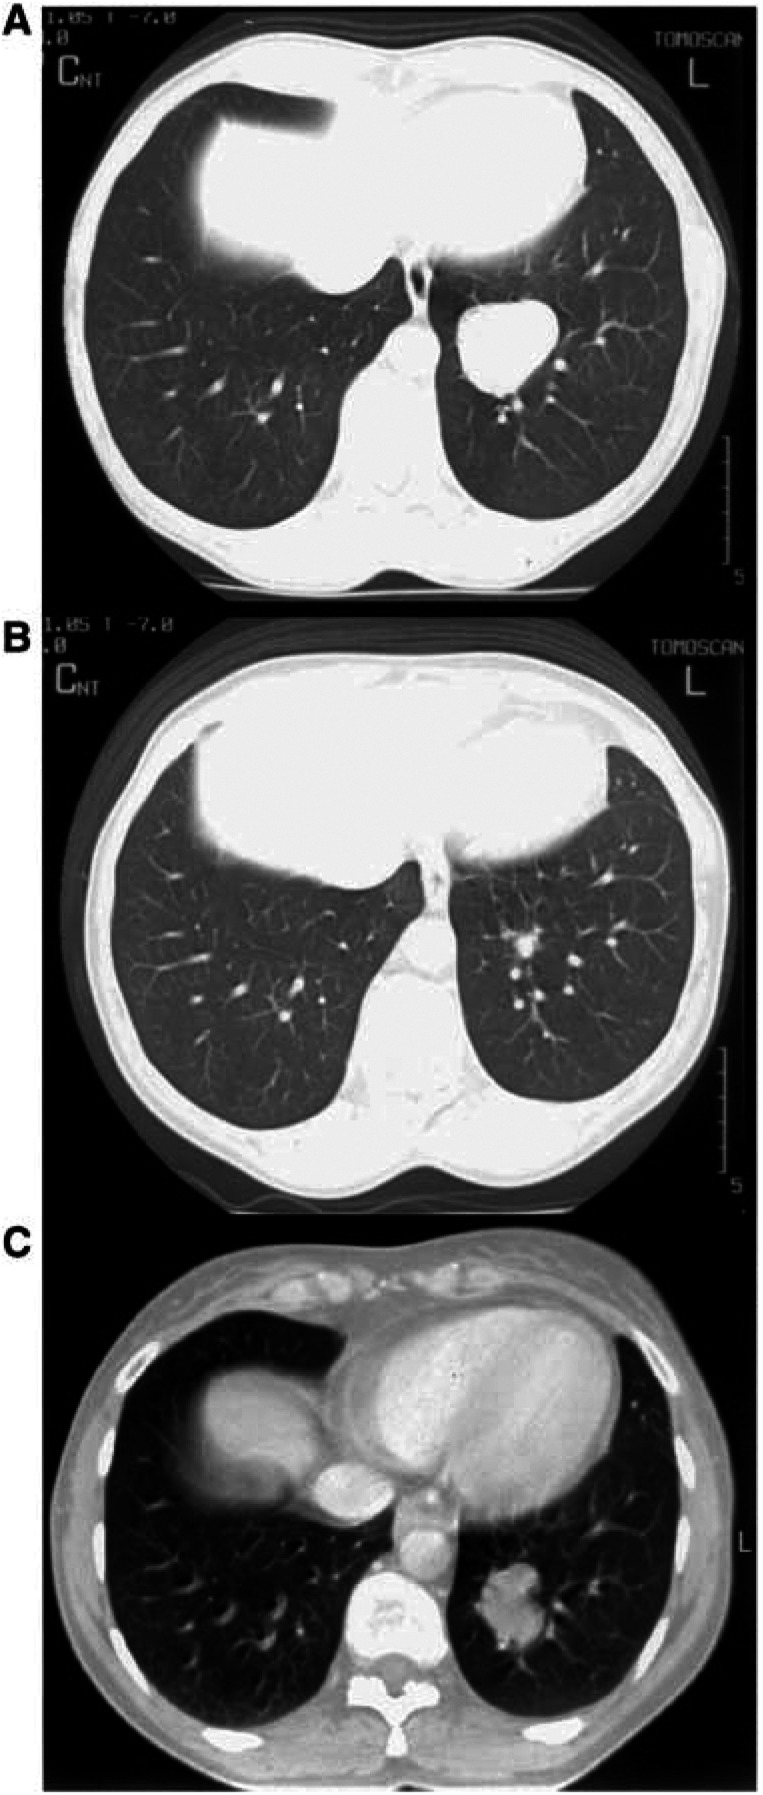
(A) CT scan of a NSCLC 78 days before induction chemotherapy; (B) CT scan made 55 days after the start of induction chemotherapy with gemcitabine and cisplatin; and (C): CT scan of the same tumour 72 days after induction chemotherapy.
